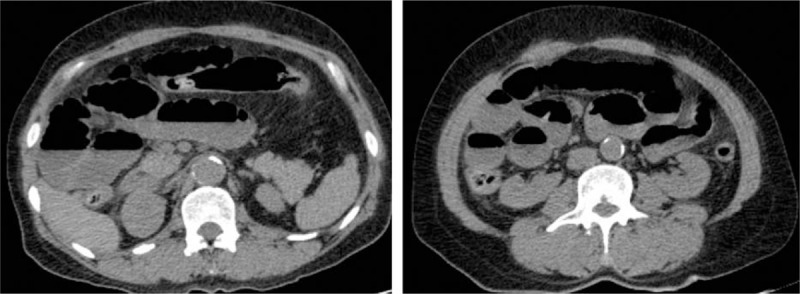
Computed tomography scan reveals small intestinal obstruction. No obvious mechanical obstruction is found on CT scanning. The small intestine is generally dilated, and air–fluid levels can be seen in the intestine. CT = computed tomography
